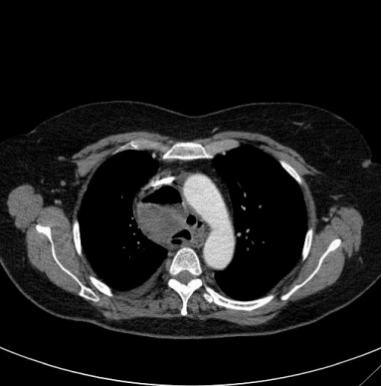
Contrast enhanced axial CT-scan of the thorax showing the cystic mass, now containing fluid, at the level of the aortic arch (mediastinal window setting).
